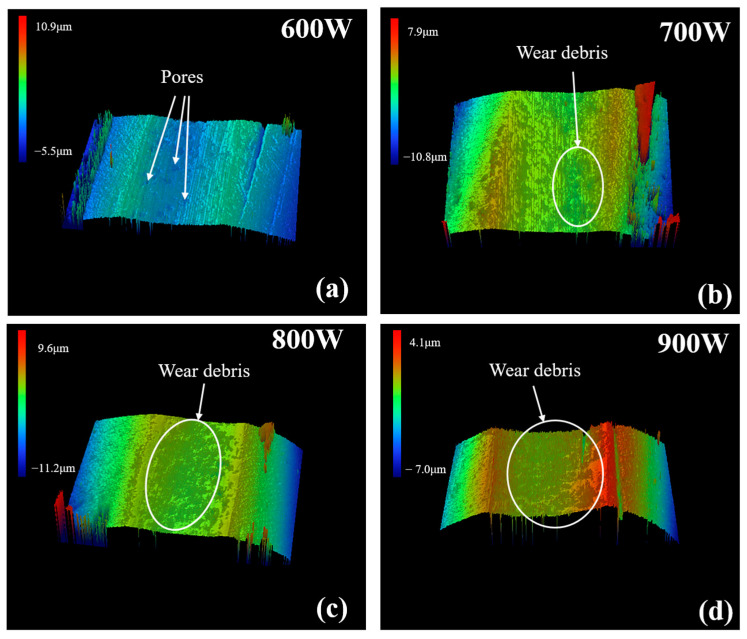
The 3D wear morphologies of coatings under different ultrasonic power: (a) 600 W; (b) 700 W; (c) 800 W; (d) 900 W.
